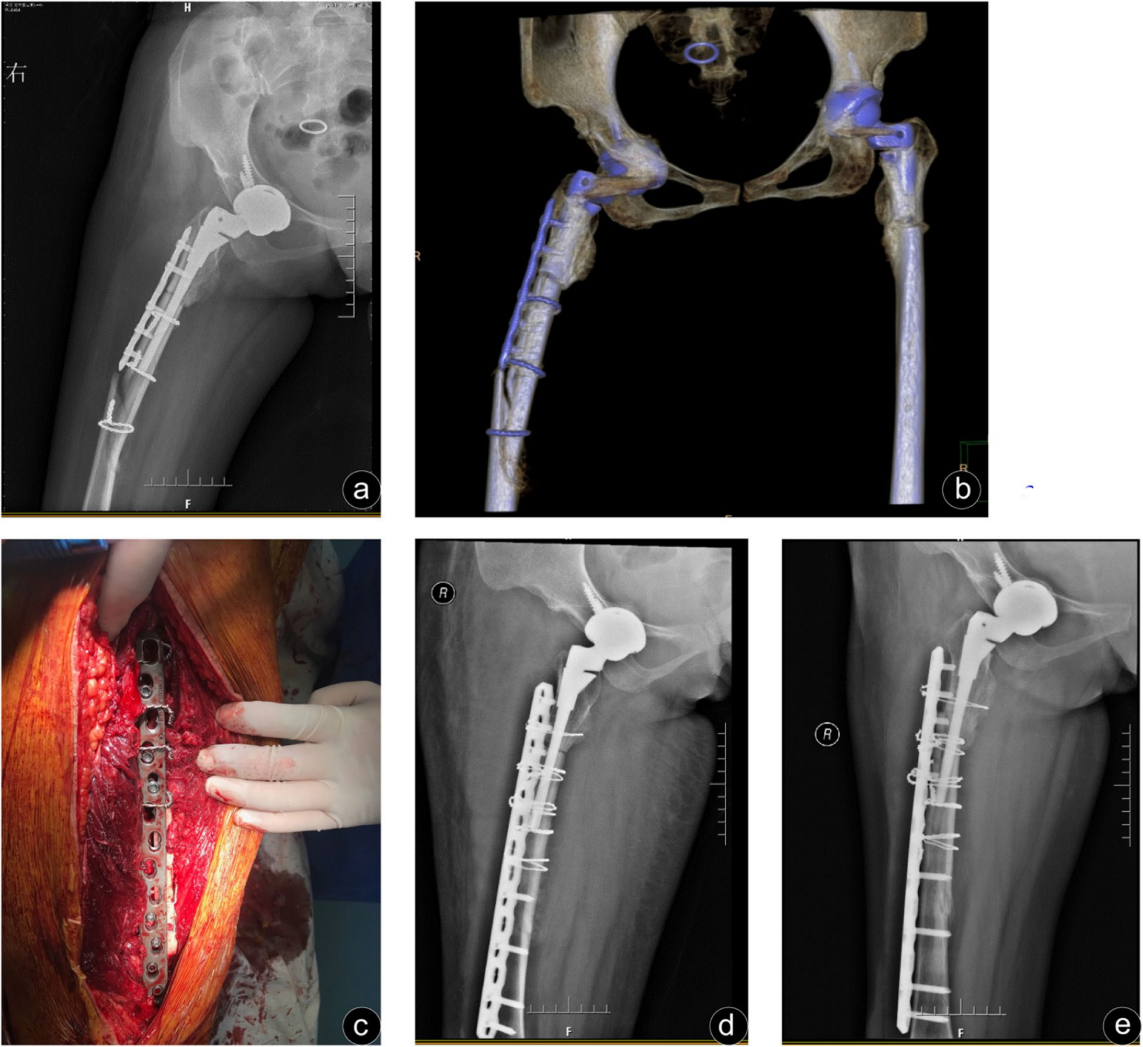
A 41-year-old female who sustained a Vancouver type B1 fracture 5 months after primary total hip arthroplasty due to Crowe type IV hip dysplasia. a and b Preoperative anteroposterior X-ray and three-dimensional reconstruction of the femur showed an oblique fracture of the distal right femoral stem. c Locked compression plate and encircling steel wire were used for fixation during operation. d The anteroposterior radiographs on the third day after the operation showed satisfactory fracture reduction, good alignment and normal pseudographs. e Anteroposterior radiographs 6 months after the operation showed distal union of the fracture, but malunion at the proximal osteotomy with a 15-degree varus deformity
